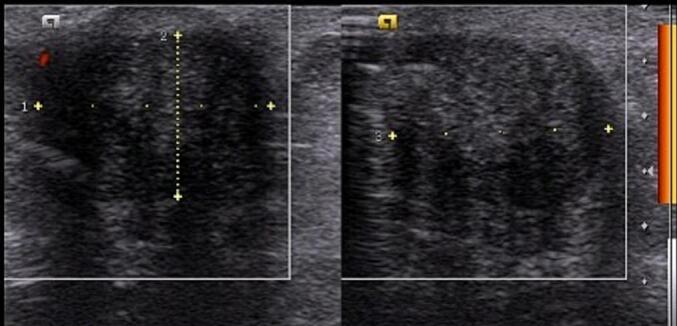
Ultrasonography examination of the second patient showed an inhomogen hypoechoic lesion, hypovascular, 2,12 × 1,46 × 1,96 cm in size with posterior shadowing in the umbilical region of the abdomen.
